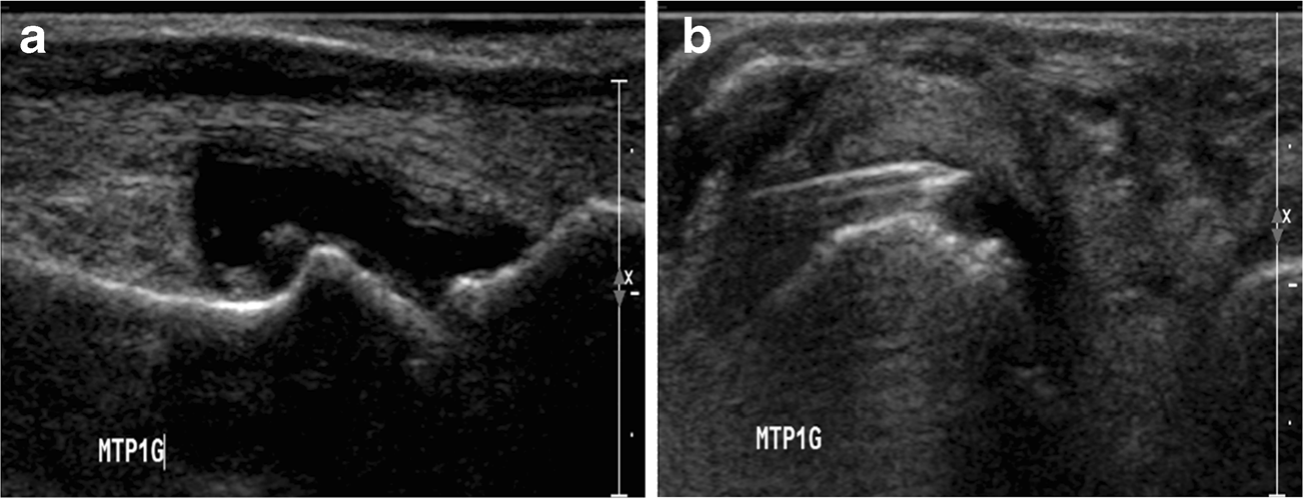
Ultrasound-guided aspiration of the first metatarsophalangeal joint. a Longitudinal view demonstrating distension of the dorsal recess by joint effusion. Note that the dorsal recess extends proximally on the dorsum of the first metacarpal. b Transverse view demonstrating the insertion of the needle in the dorsal recess and fluid aspiration
